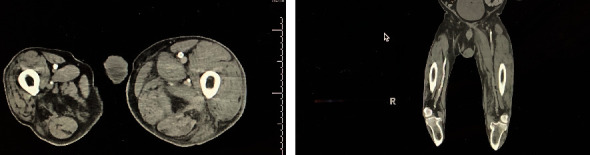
Computed tomography angiography showing the lack of arterial blood flow in the left superficial femoral and proximal popliteal arteries.
